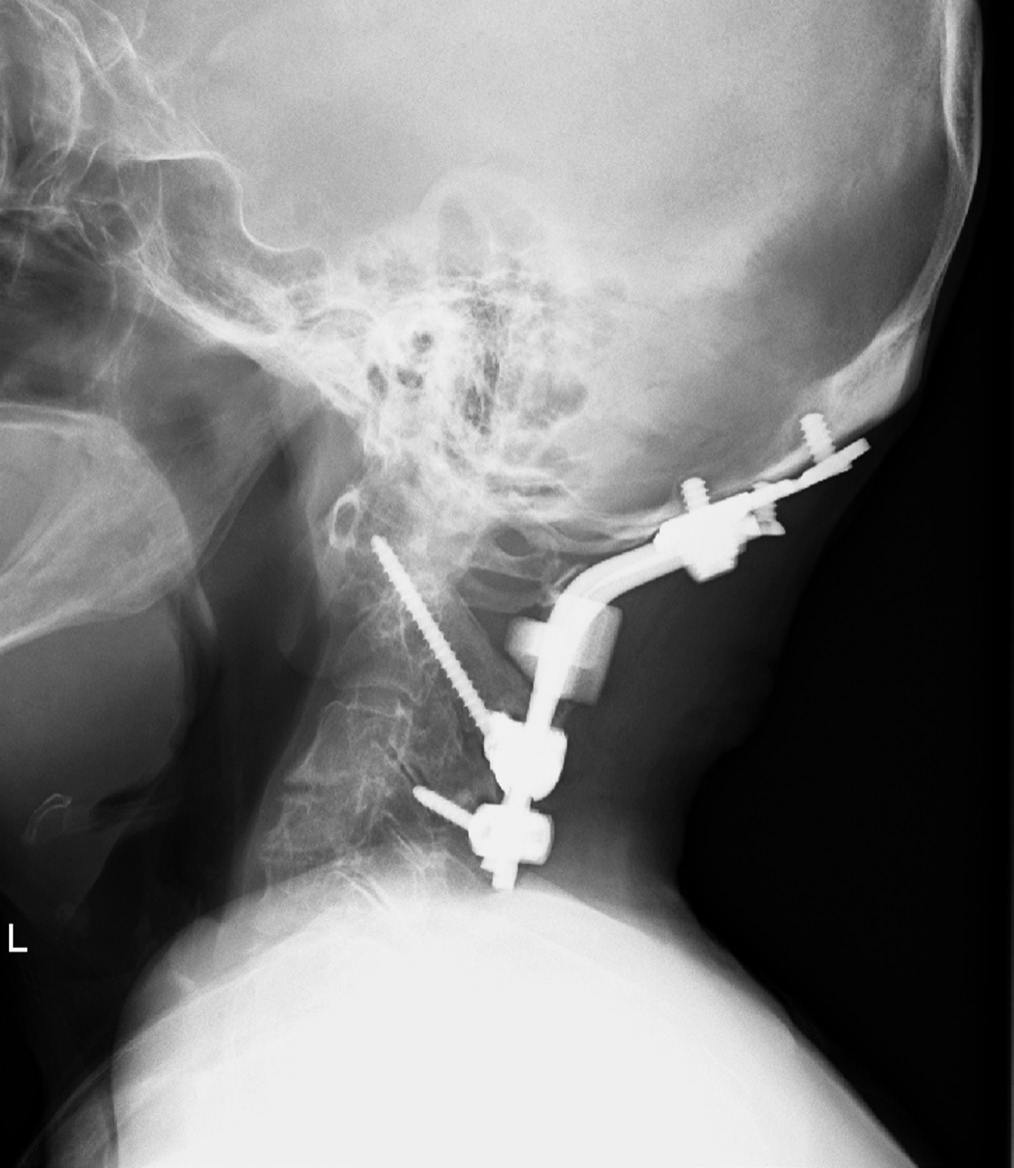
Postoperative plain radiograph of the lateral cervical spine showing fusion of the fracture and no evidence of instrumentation loosening.
